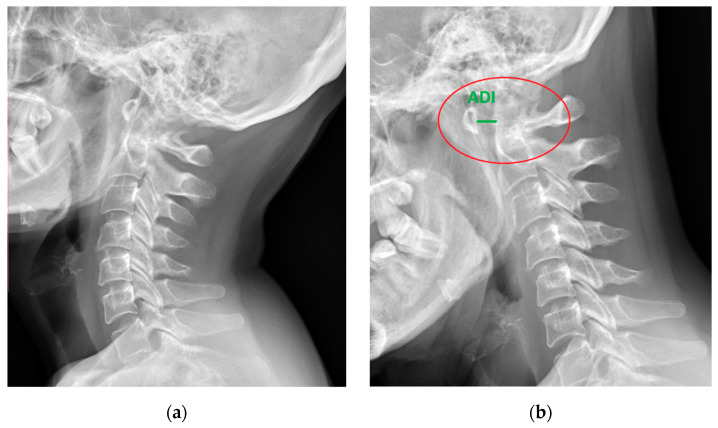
Lateral X-ray of the neck of a 49-year-old patient with rheumatoid arthritis and atlantoaxial subluxation (red circle), (a) neutral position, and (b) flexion. ADI—atlantodental interval (green).
